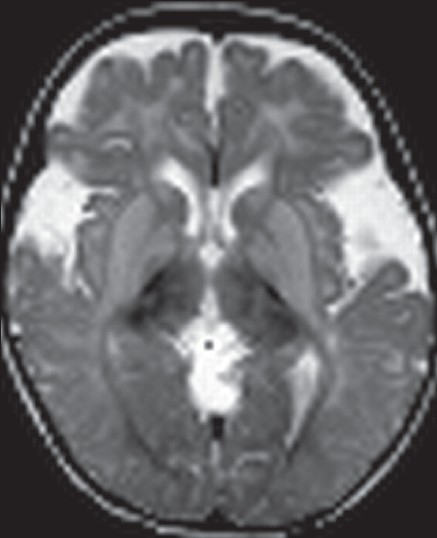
MRI (T2 W) in a 15 months old baby with glutaric aciduria type 1. Note the widened sylvian fissures, bilateral symmetrical signal changes in the basal ganglia. and widened subarachnoid spaces
